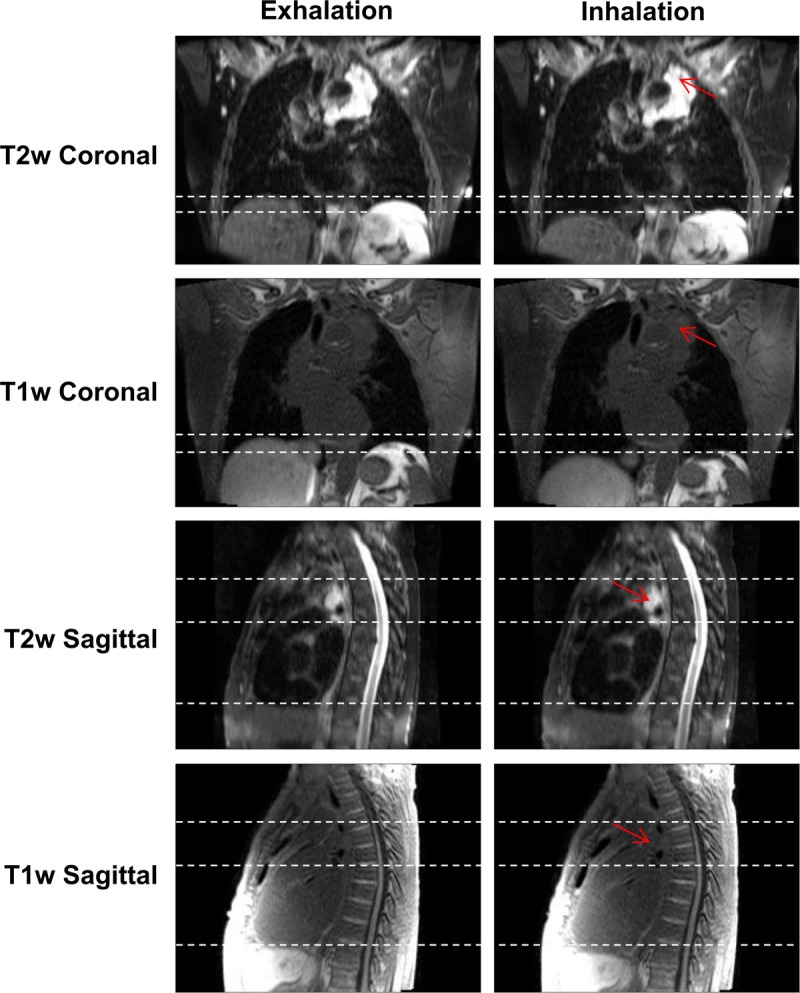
Snapshots of 4D-T1w and 4D-T2w MRI at the exhalation and inhalation respiratory phases for patient 3, who was diagnosed with T4N2 adenocarcinoma. The tumor is radically treatable, but is embedded around the heart and oesophagus. Unlike T1w MRI, T2w MRI displays a high tumor-tissue contrast enabling tumor position and structure to be clearly delineated. Sliding motion of the tumor site against the chest wall is displayed in the sagittal plane and is more clearly presented in T2w than T1w MRI. Red arrows point to the tumor site and dashed white lines aid assessment of both superior-inferior diaphragm and sliding motion.
